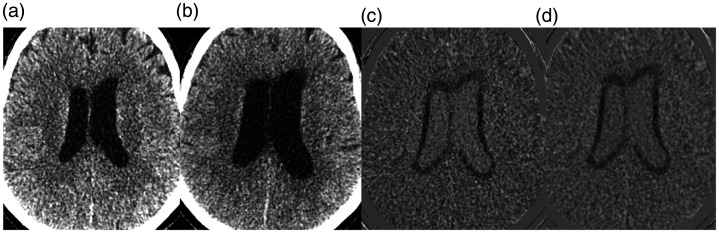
a, Baseline investigation for ventricular size estimation on nonenhanced computed tomography (CT) with (b) follow-up images on nonenhanced CT and (c) pseudosubtraction of the two volumes in GE AW server and (d) Philips IntelliSpace Portal. As shown in (c) to (d), the dark rim around the ventricles represents their size progression. Surrounding stationary findings are grey due to the inverse relationship between the overlaid three-dimensional volumes (volume (a) inverse grey, volume (b) greyscale). GE AW: General electric healthcare advanced workstation.
